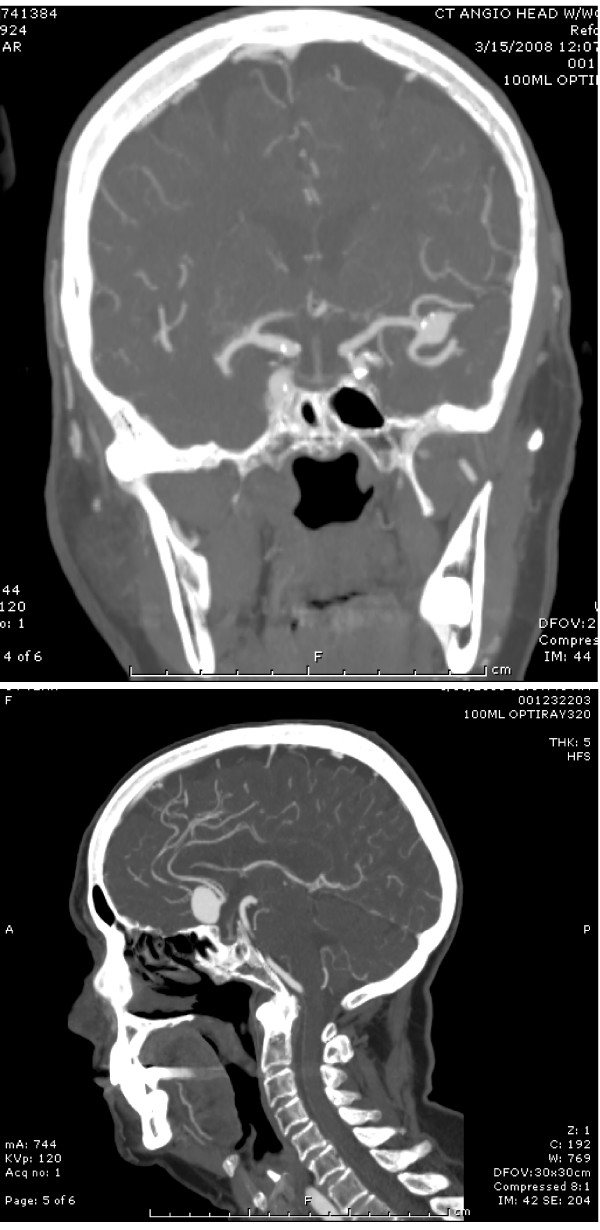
Anteroposterior (a) and lateral (b) views of CT angiogram. These images demonstrate large middle cerebral artery aneurysm and giant anterior communicating artery aneurysm.
